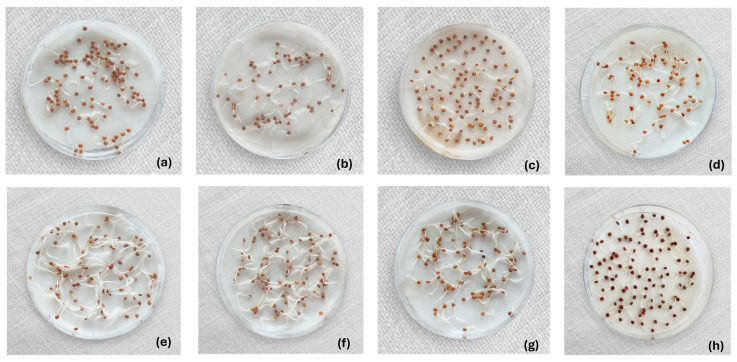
Visual comparison of Pasankalla (PK) quinoa grain germination after seven disinfection treatments: (a) 4% acetic acid; (b) 200 ppm sodium hypochlorite; (c) 70% alcohol (d) 8% hydrogen peroxide; (e) ultrasound 15 min + 200 ppm sodium hypochlorite; (f) ultrasound 30 min + 200 ppm sodium hypochlorite; (g) water 50 °C + 2% hydrogen peroxide + acetic acid 0.1%; (h) control.
